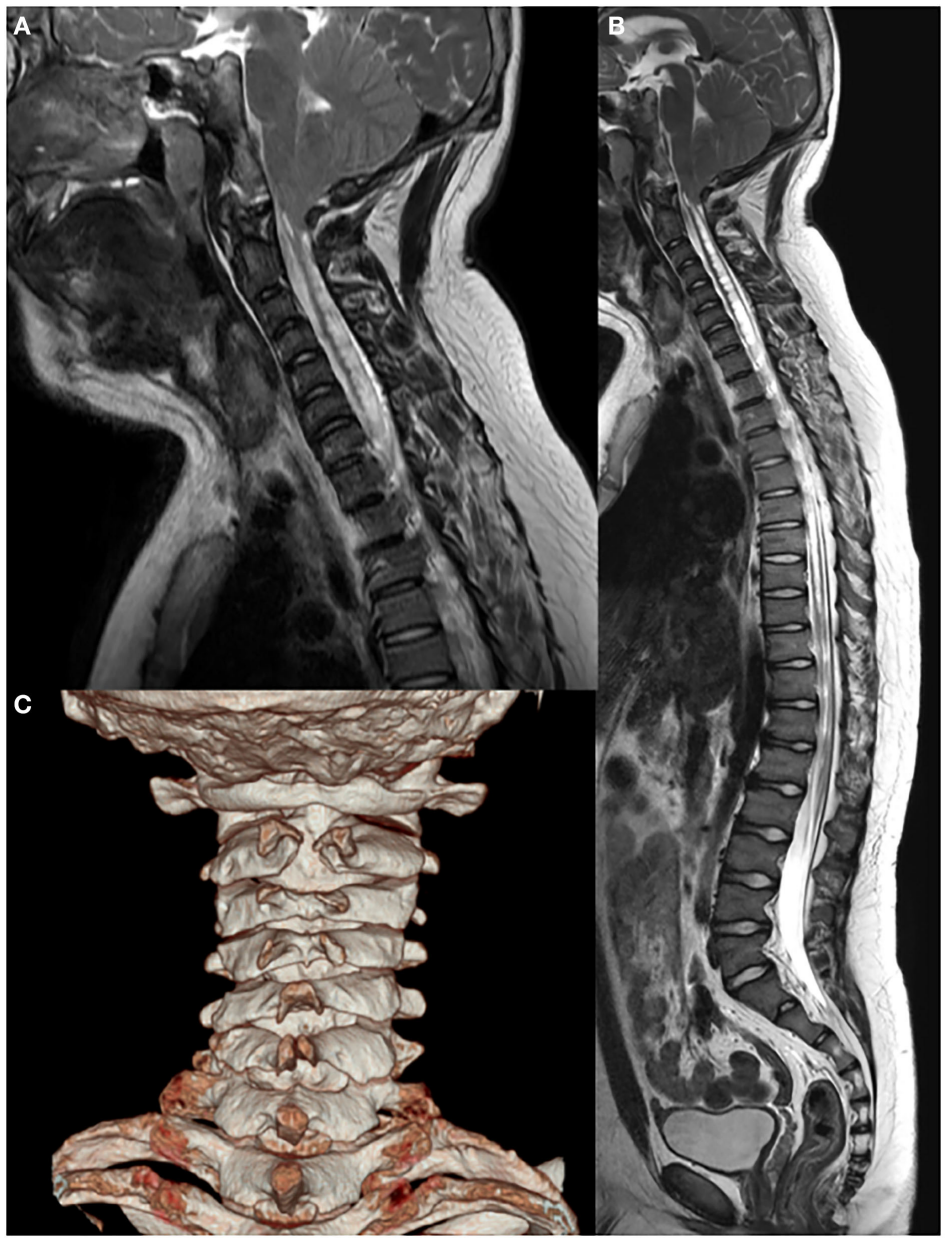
Brain and whole-spine magnetic resonance imaging and computed tomography showing (A,B) Chiari I malformation with extensive hydrosyringomyelia from the C1 to T12 levels and (C) C2 spina bifida.
